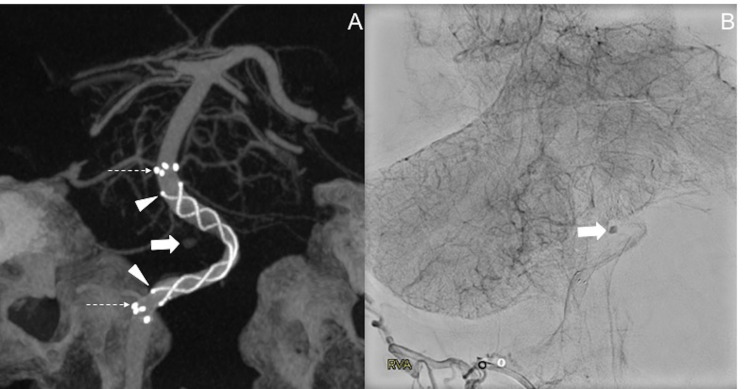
Endovascular treatment of the ruptured basilar perforator aneurysm. Three-dimensional reconstruction of a Vaso-CT (A) performed after placement of the Flow Re-Direction Endoluminal Device (FRED) shows the distal device markers indicating the end of the flare tips of the device (dotted arrow), the central flow diversion portion of the device (arrowheads), and the location of the perforator aneurysm (thick arrow). Delayed images of the angiogram post device deployment (B) show contrast stagnation within the basilar artery perforator aneurysm.
